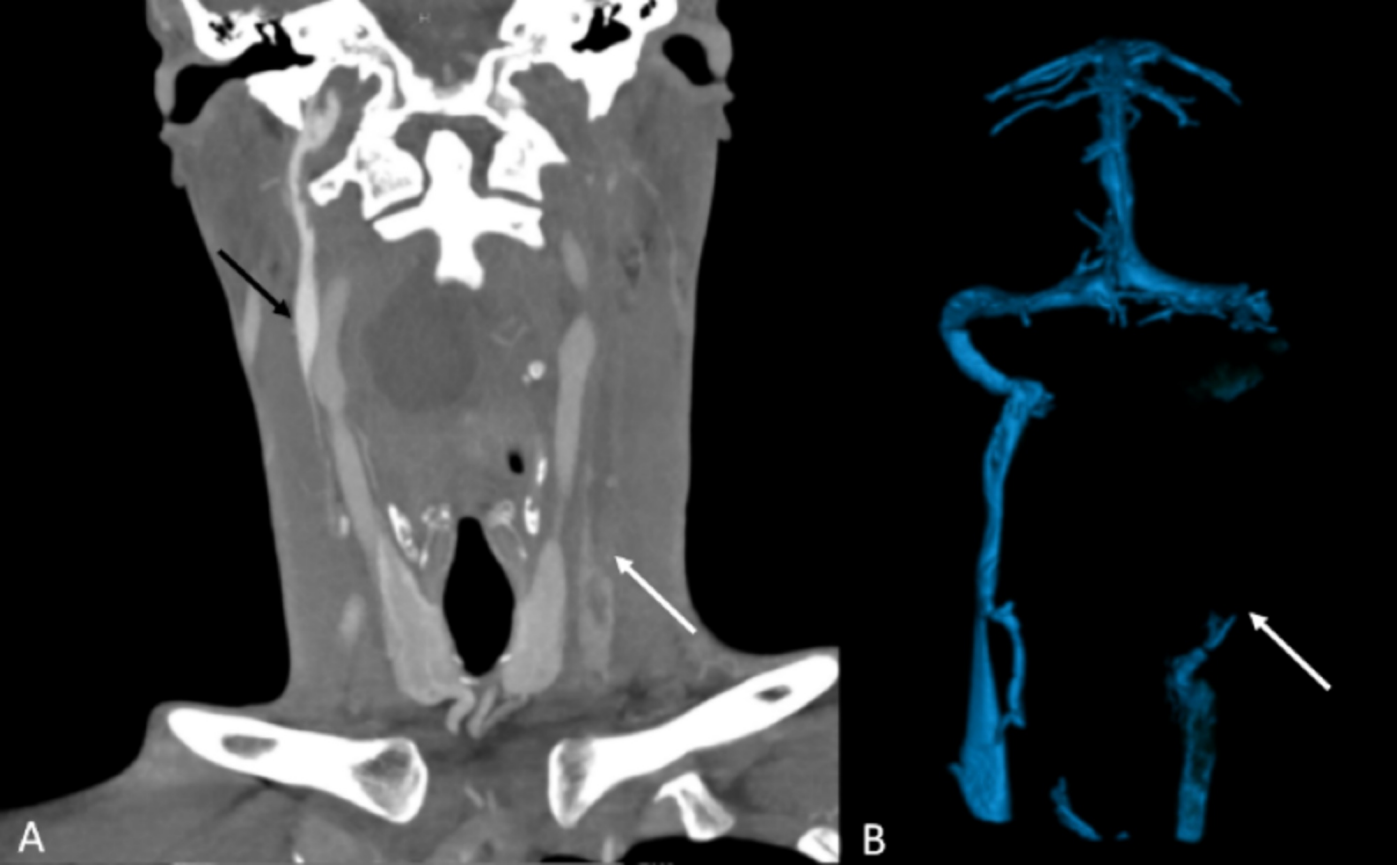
Coronal CT angiography of the neck and 3D CT reconstruction of the venous system (A) Coronal CT angiography of the neck shows complete occlusion of the left jugular vein with thrombus extending cephalad to the sigmoid sinus (white arrow) and patent right IJV (black arrow), (B) 3D CT reconstruction of the venous system demonstrates complete occlusion of the left IJV (white arrow). IJV - internal jugular vein
